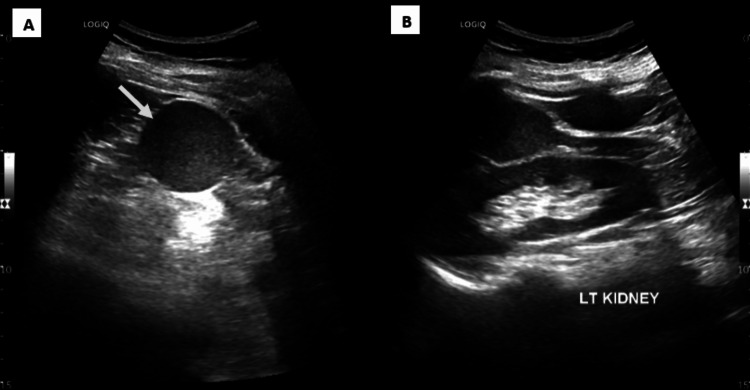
Ultrasonography of the abdomen (A) Absence of the native spleen in the splenic fossa, consistent with prior splenectomy, with a well-defined lesion (arrow) identified in the splenic fossa. (B) The same lesion (arrow) visualized adjacent to the upper pole of the left kidney, demonstrating homogeneous echogenicity comparable to splenic tissue, suggestive of solitary splenosis.
